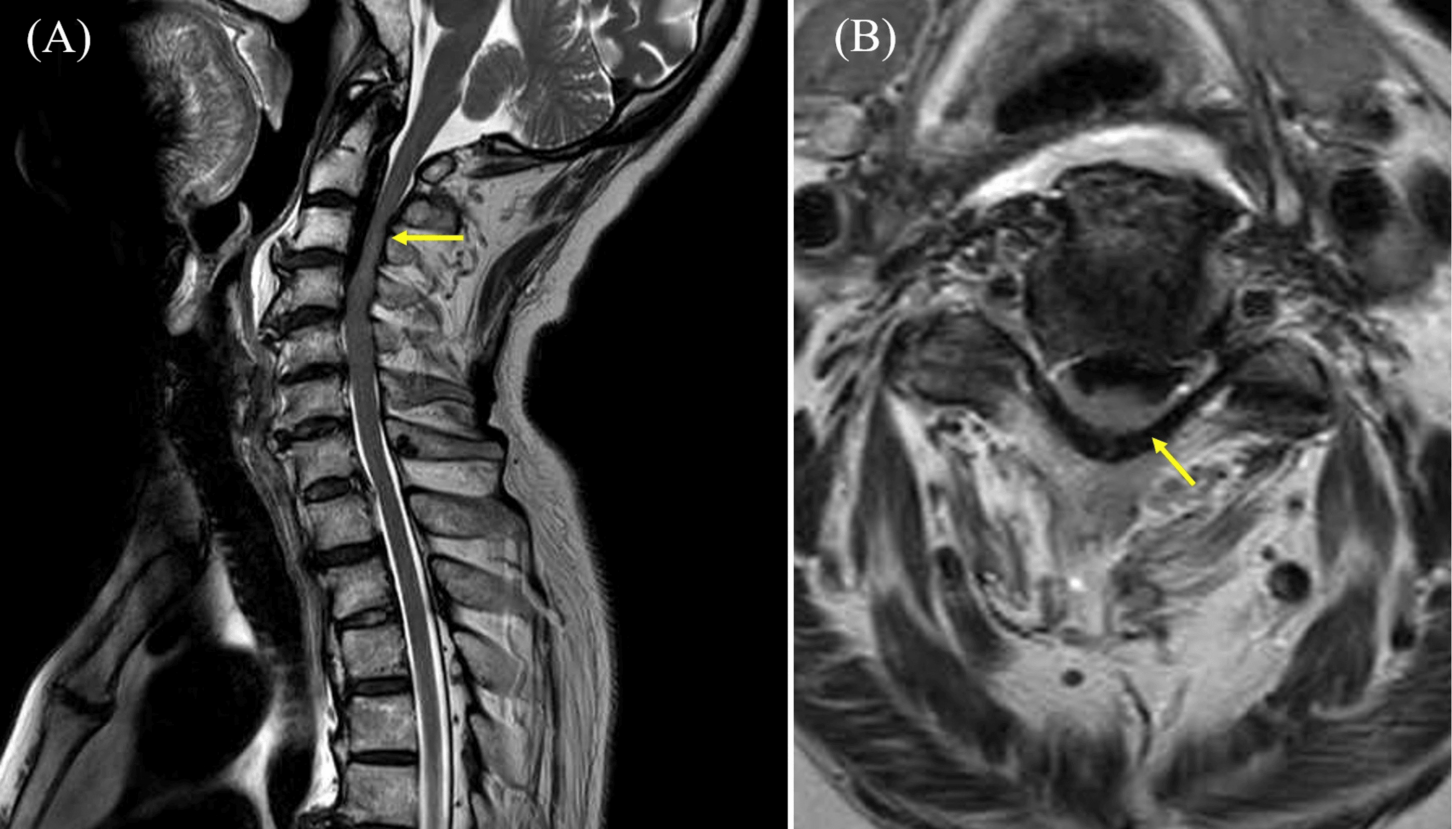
Cervical MRI findings on T2-weighted images. (A) Sagittal MRI of the cervical spine showing severe stenosis at C2-4, moderate stenosis at C4-6, and intramedullary T2 hyperintensity at the same levels (arrow). (B) Axial MRI at the C2 level demonstrating severe stenosis and hyperintensity of the cervical spinal cord (arrow). MRI, magnetic resonance imaging.
